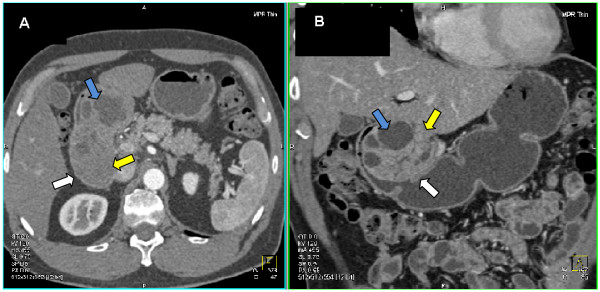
Appearance of a Giant Brunner's Gland Hamartoma on Computed Tomography Scan. (a) Contrast enhanced axial image in arterial phase of acquisition demonstrates an approximately 10 cm mass in duodenum extending into the gastric antrum (white arrow) with both solid (yellow arrow) and cystic components (blue arrow) (b) coronal display shows the intraluminal nature of the mass (white arrow) and its extent as well as again defining both the solid (yellow arrow) and cystic components (blue arrow).
